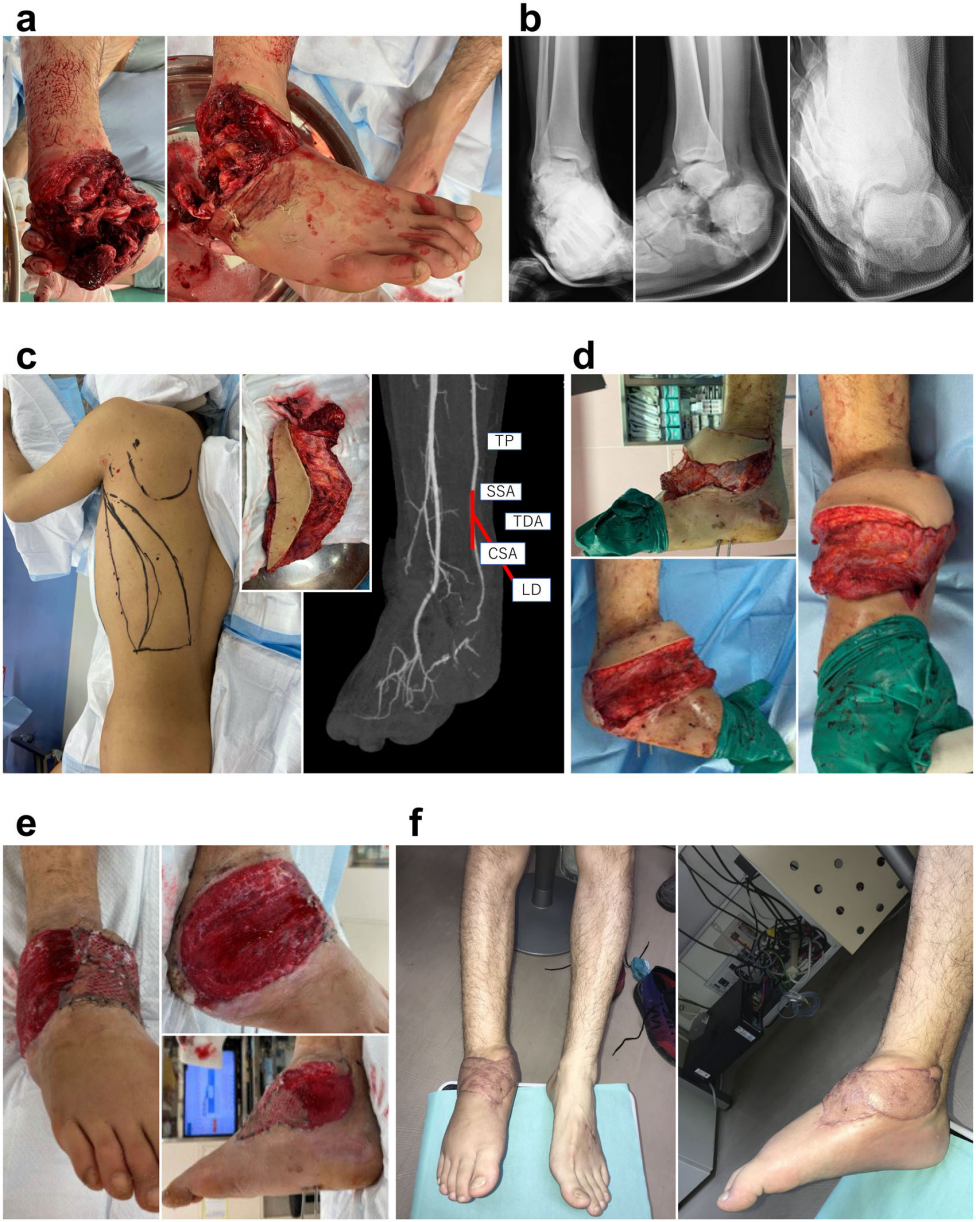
Patient 1. (A) Upon arrival at the emergency room, the blood circulation in the right foot was poor. (B) Multiple fractures and dislocations were observed after performing radiographic examination. (C) The recipient artery was interposed with T portion and the flow was sutured through anastomosis to the TP artery. (D) On day 9, free LD flap was performed. Abbreviation: (E) After the third tangential excision (4 weeks after LD flap). (F) Appearance at 3.5 months after injury. TP: tibialis posterior artery; SSA: subscapular artery; CSA: circumflex scapular artery; TDA: thoracodorsal artery; LD: latissimus dorsi.
